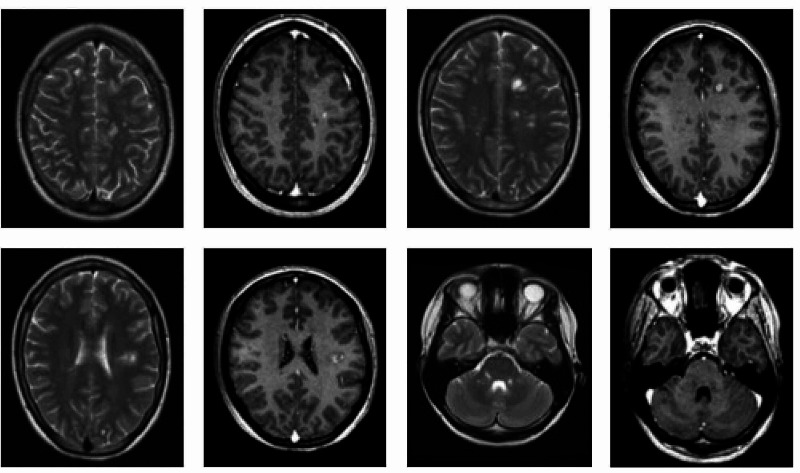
MRI brain - 12 weeks post injury; T2 weighted and T1 weighted with contrast at different axial cuts
